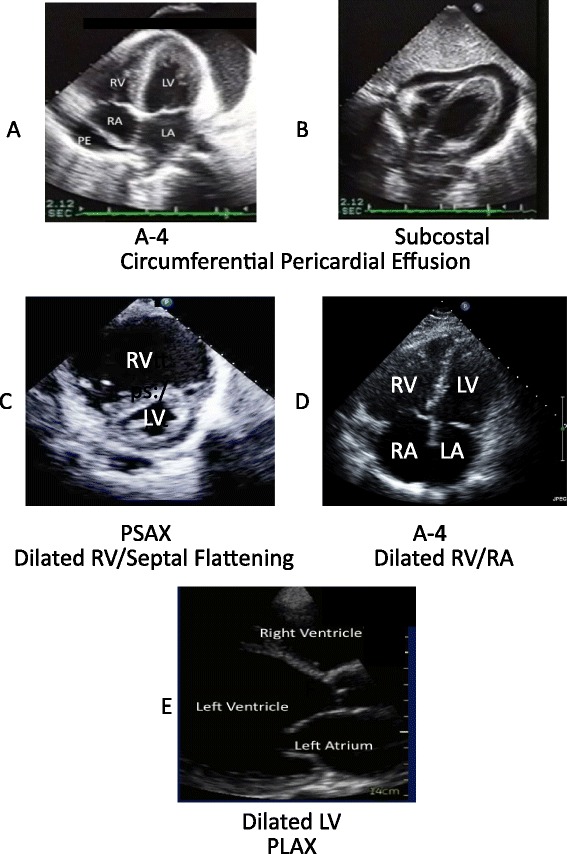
Examples of pathological findings. (A) Apical four chamber (A-4) view. Circumferential pericardial effusion. Left ventricle (LV), right ventricle (RV), left atrium (LA), right atrium (RA), and pericardium with a circumferential echocardiographic-free space or pericardial effusion (PE). (B) Subcostal view. LV, RV, LA, RA, and pericardium with a circumferential pericardial effusion. Diastolic right ventricular ‘buckling’ or collapse is demonstrated. (C) Parasternal short-axis view (PSAX) of the RV and the LV. The RV is dilated. The interventricular septum demonstrates ‘flattening’ or ‘displacement’, indicating evidence of RV pressure or volume overload. (D) A-4 view. Dilated RV, dilated RA, LV, and LA. (E) Parasternal long axis (PLAX) view of the RV, dilated LV, aorta, and LA.
